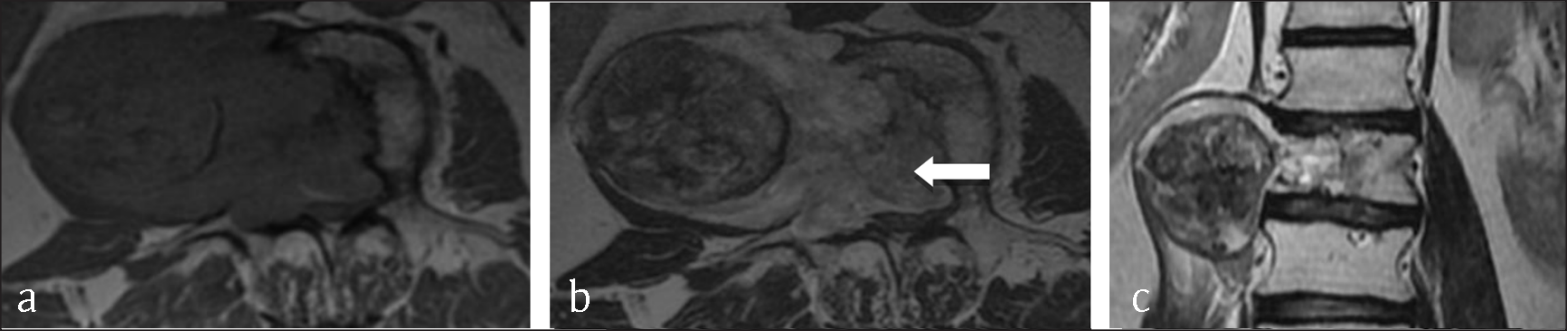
Chordoma of L2. MR. (a) Axial T1-weighted, (b) axial T1-WI after gadolinium contrast injection, coronal T2-WI (c) show a lobulated mass lesion of heterogeneous signal originating from the vertebral body of L2 with large soft tissue component extending into the paravertebral muscles (curved arrow) and anterior epidural space (arrow).
